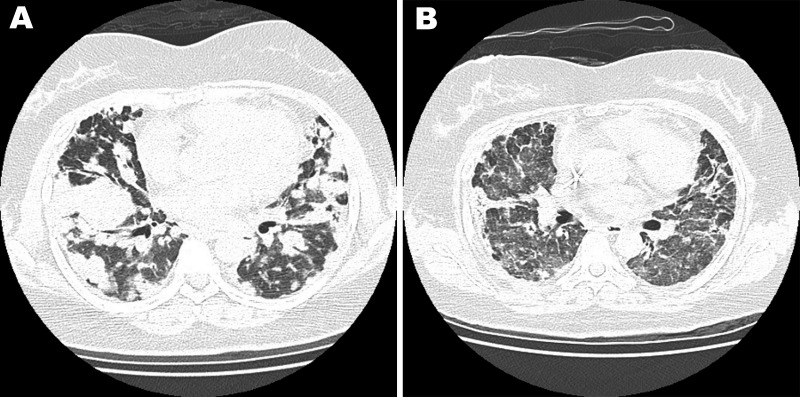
Chest computed tomography scan of a 40-year-old woman with no history of systemic disease. A) Bilateral multiple lung nodules and lymphomatoid granulomatosis were diagnosed after lung biopsy. B) After rituximab treatment, the prior nodular lung lesions decreased dramatically, but newly developed interstitial ground glass opacities appeared.
